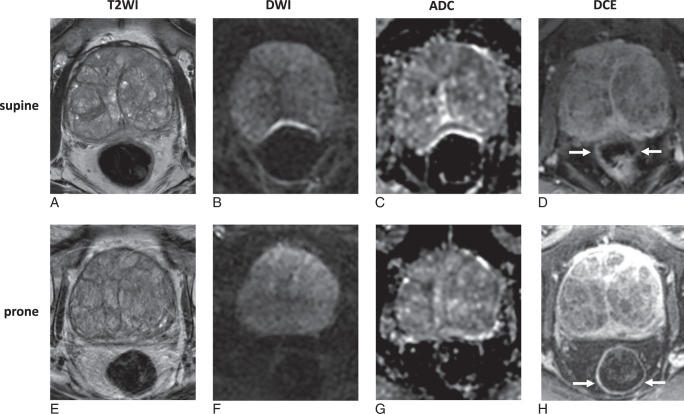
Prostate MR images from the same patient demonstrating improved image quality of prone DWI (F) and ADC map (G) compared with supine DWI (B) and ADC map (C). However, supine T2WI (A) showed superior image quality to prone T2WI, which demonstrates motion artefact (E). DCE sequence better demonstrates the anterior location of air at the rectoprostatic interface (arrows) on supine imaging (D) and the more posterior location (arrows) on supine imaging (H). ADC indicates apparent diffusion coefficient; DCE, dynamic contrast-enhanced T1-weighted imaging; DWI, diffusion-weighted imaging; T2WI, T2-weighted imaging.
